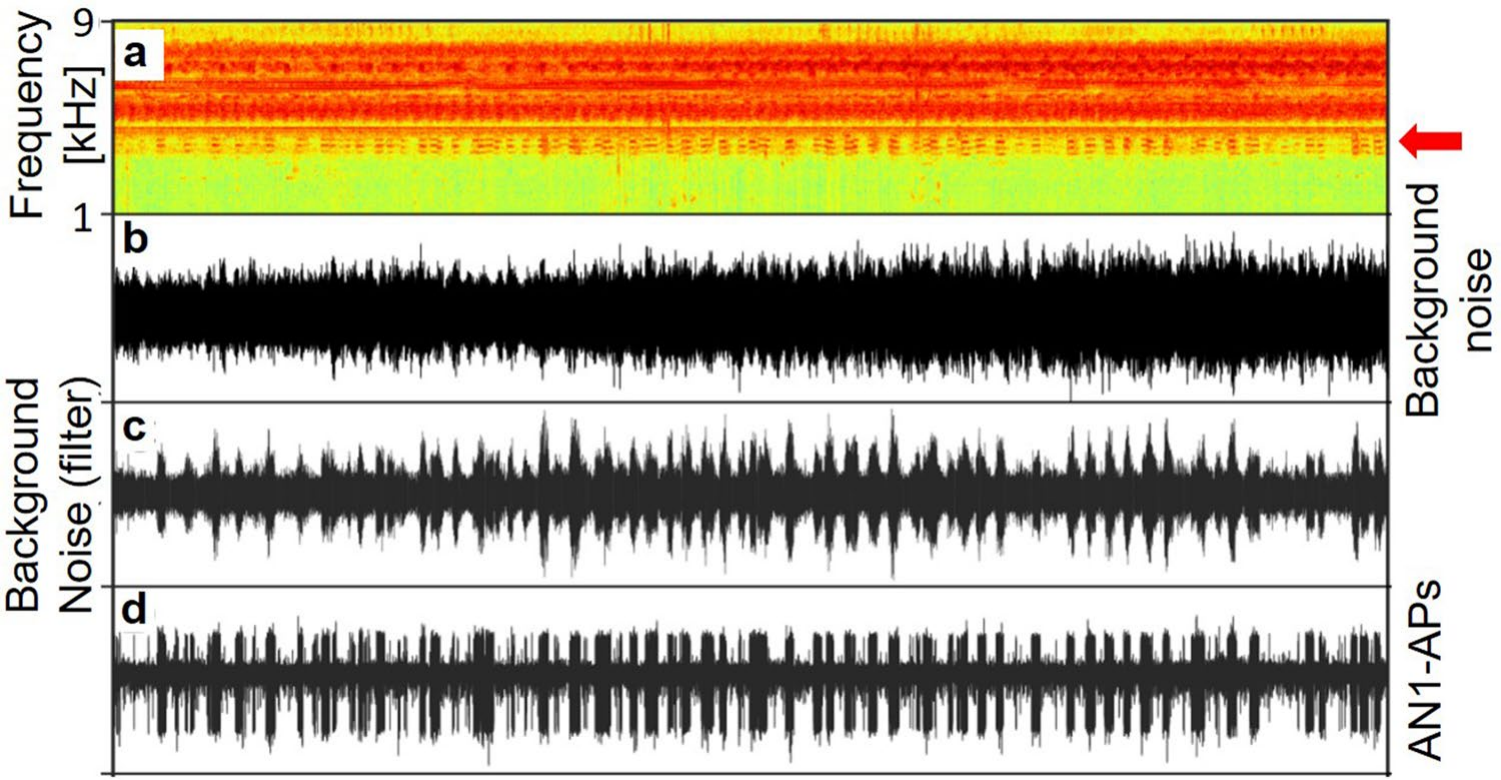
Representative section of 30 s of nocturnal background noise recording as sonogram and oscillogram, respectively (a, b). Filtering of this sound section with a filter function derived from the tuning curve of AN1 in *Diatrypa* sp*.* (c) reveals an amplitude modulation coinciding with the bursting activity of the AN1 neuron (d). Bursts were elicited by sound events in the narrow frequency band between 3.5 and 4.5 kHz, representing calling songs of several *Diatrypa* males at various distances from the preparation (arrow in a). Modified from Schmidt et al. (2011)
